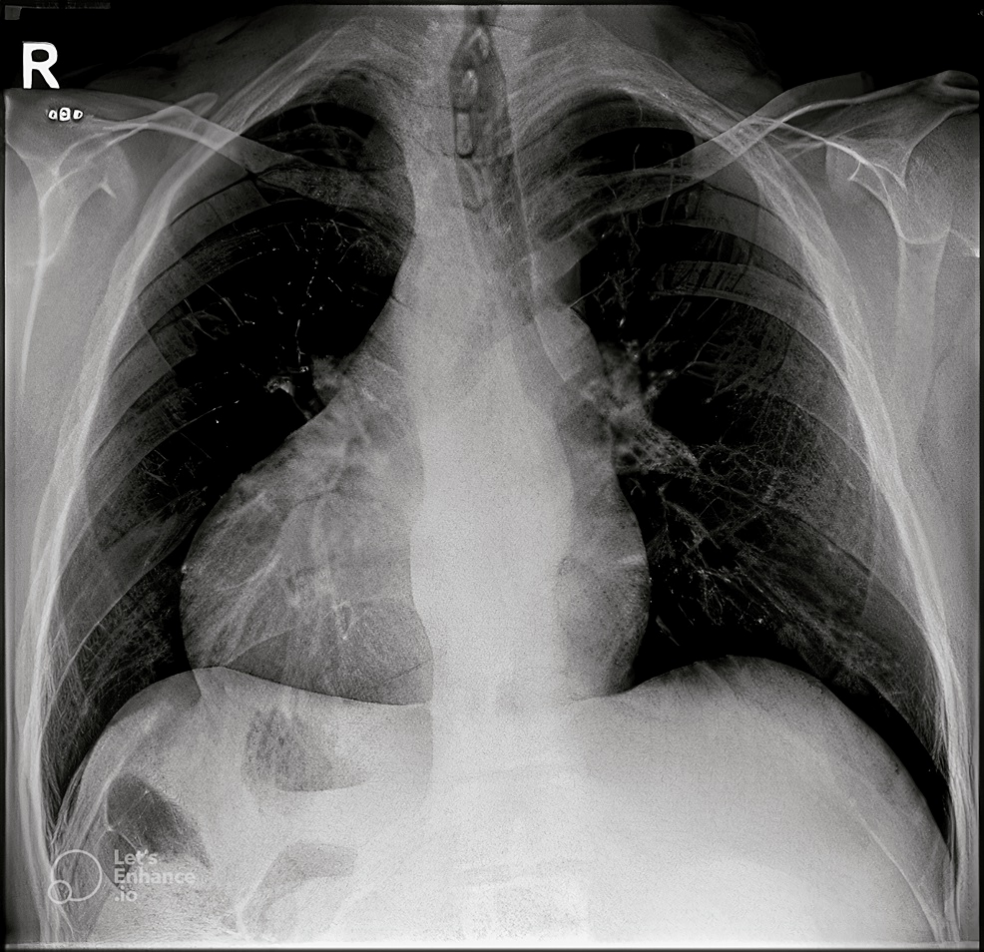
Chest X-ray showing dextrocardia with air fluids levels in the right upper quadrant of the abdomen
